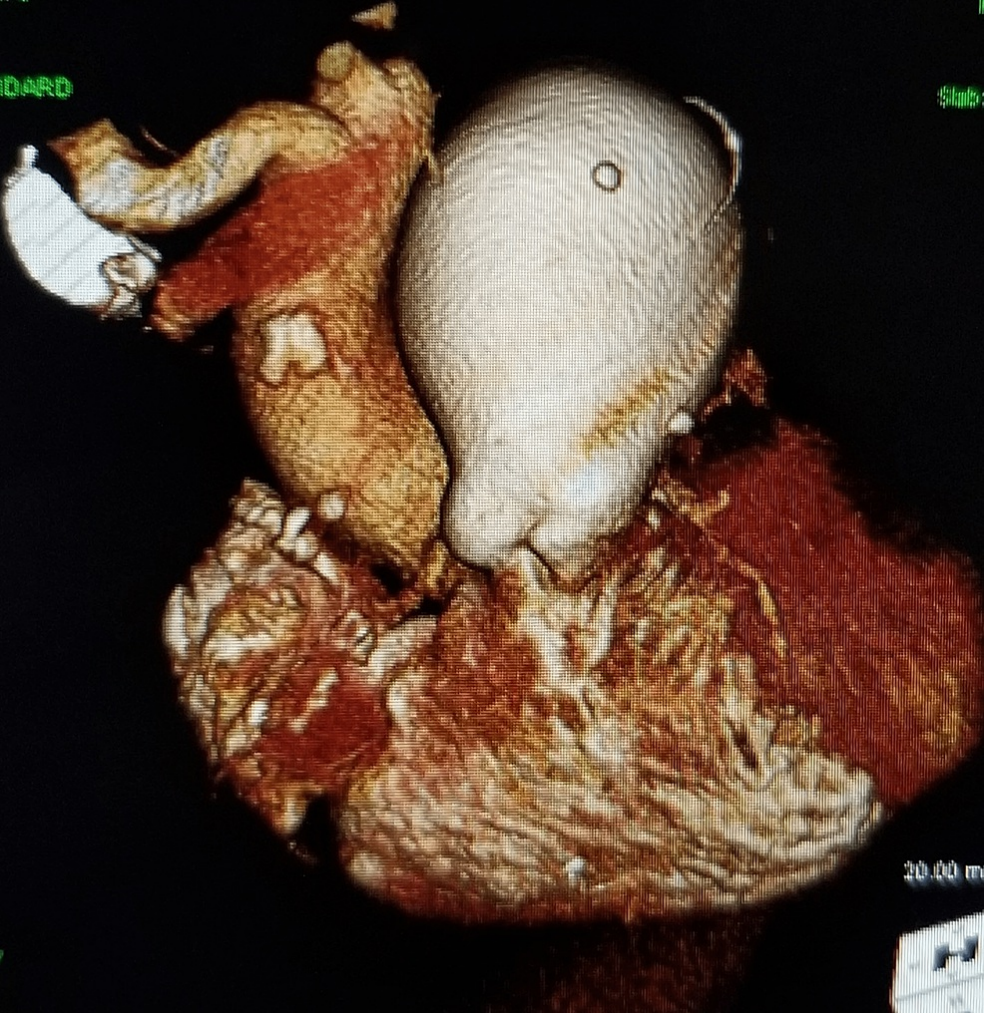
PAA as seen on 3D reconstruction CTA
